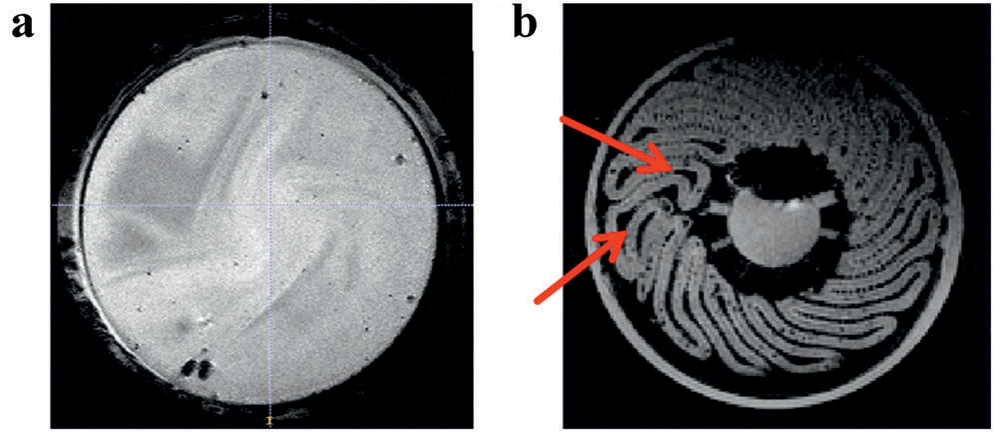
a: Cross-sectional MRI scan through the center of the membrane stack of an axial flow XT5 capsule that has been cleaned using 1 N NaOH, (b): Cross-sectional MRI scan of the XT140 capsule. The membrane pleats are clearly visible in gray, due to their water content.
